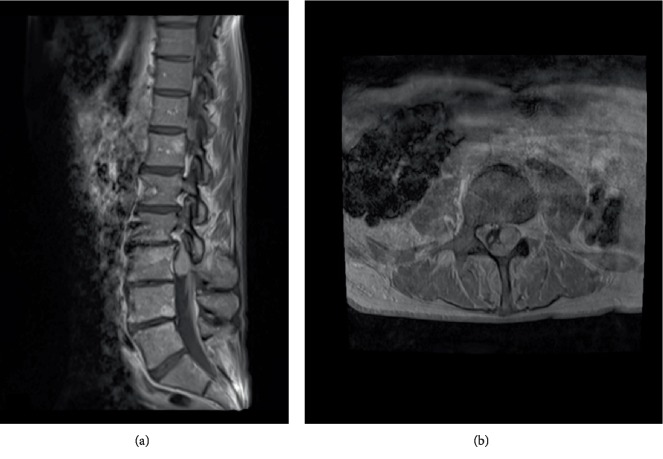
(a) Magnetic resonance imaging (MRI) T1-weighted sagittal image of the lumbar spine with gadolinium demonstrates a well demarcated intradural extramedullary mass. (b) Corresponding axial image.
